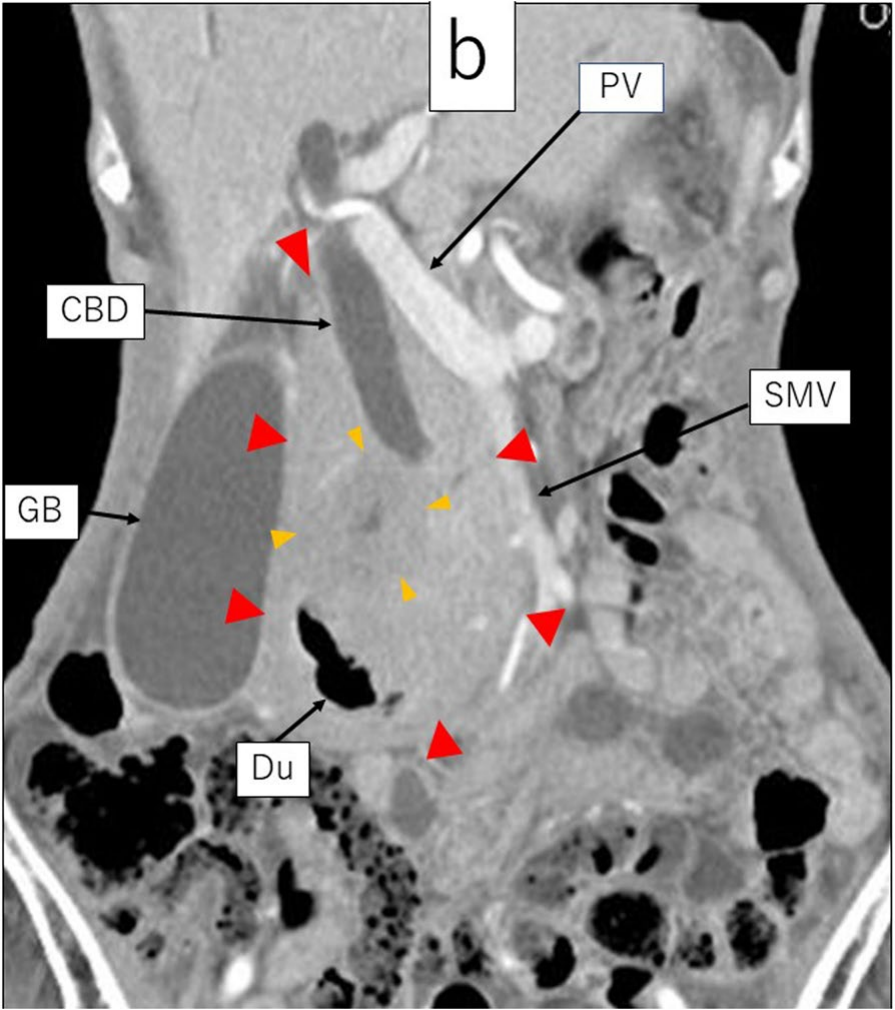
The early phase of contrast-enhanced CT (coronal view); A large homogeneous low-density lesion (
